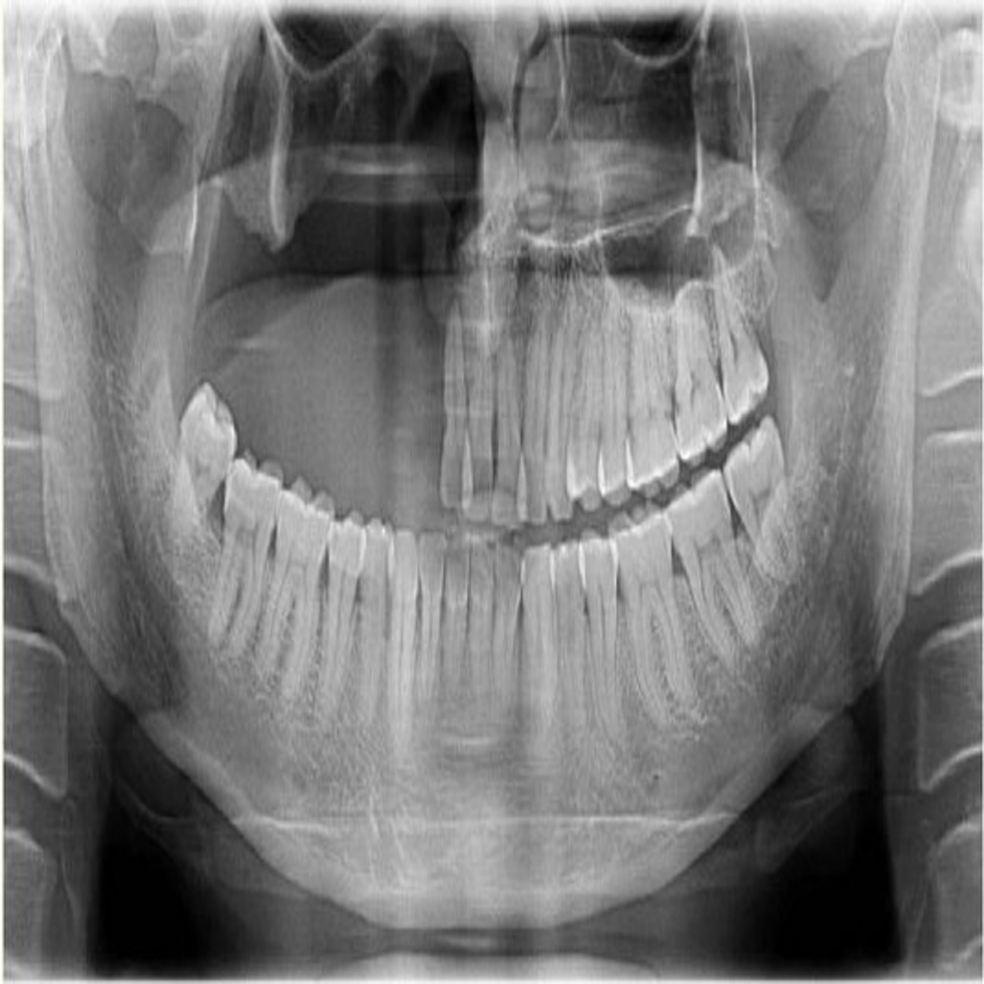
Orthopantomagram revealing the residual bone and intraoral status
